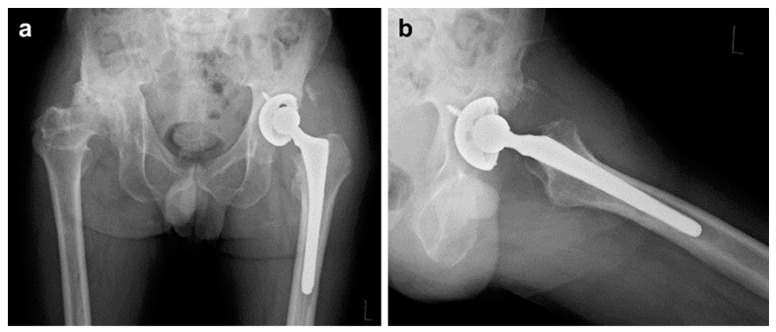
Four months after the primary surgery, cup loosening and displacement were observed. (a) An anteroposterior plain radiograph of the bilateral hips at 4 months after primary surgery. (b) Axial image radiograph of the left hip at 4 months after primary surgery.
